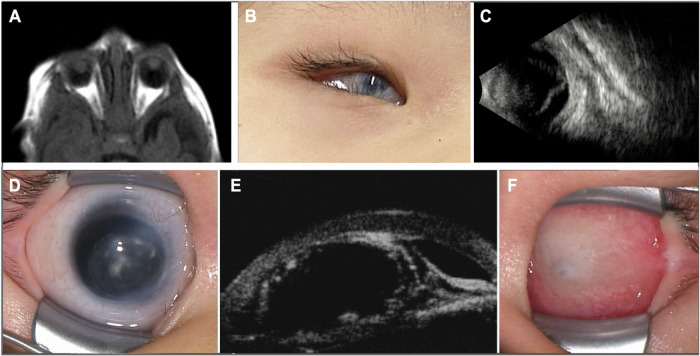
Ocular imaging findings of the two cases. A Magnetic resonance imaging of both eyes of case 1, acquired using a T1-weighted fluid-attenuated inversion recovery sequence. B Anterior segment photograph of the right eye in case 1. The patient exhibited bilateral microphthalmia, corneal opacity, anterior segment dysgenesis and left chorioretinal coloboma. The corneal diameters were approximately 5.0 mm in both eyes. C The left eye developed retinal detachment, which B-mode ultrasonography confirmed, suggesting a rhegmatogenous etiology potentially due to self-inflicted ocular pressure. D Anterior segment photograph of the right eye of case 2. E B-mode ultrasonography of the right eye of case 2. The initial ophthalmologic examination at 6 weeks of age revealed Peters anomaly type II in the right eye, characterized by central corneal opacity with iridocorneal and lenticulocorneal adhesions. The corneal diameter measured 9 × 9 mm in the right eye. F The left eye of case 2 exhibited extreme microphthalmia. The corneal diameter measured 1.5 × 1.5 mm in the left eye.
